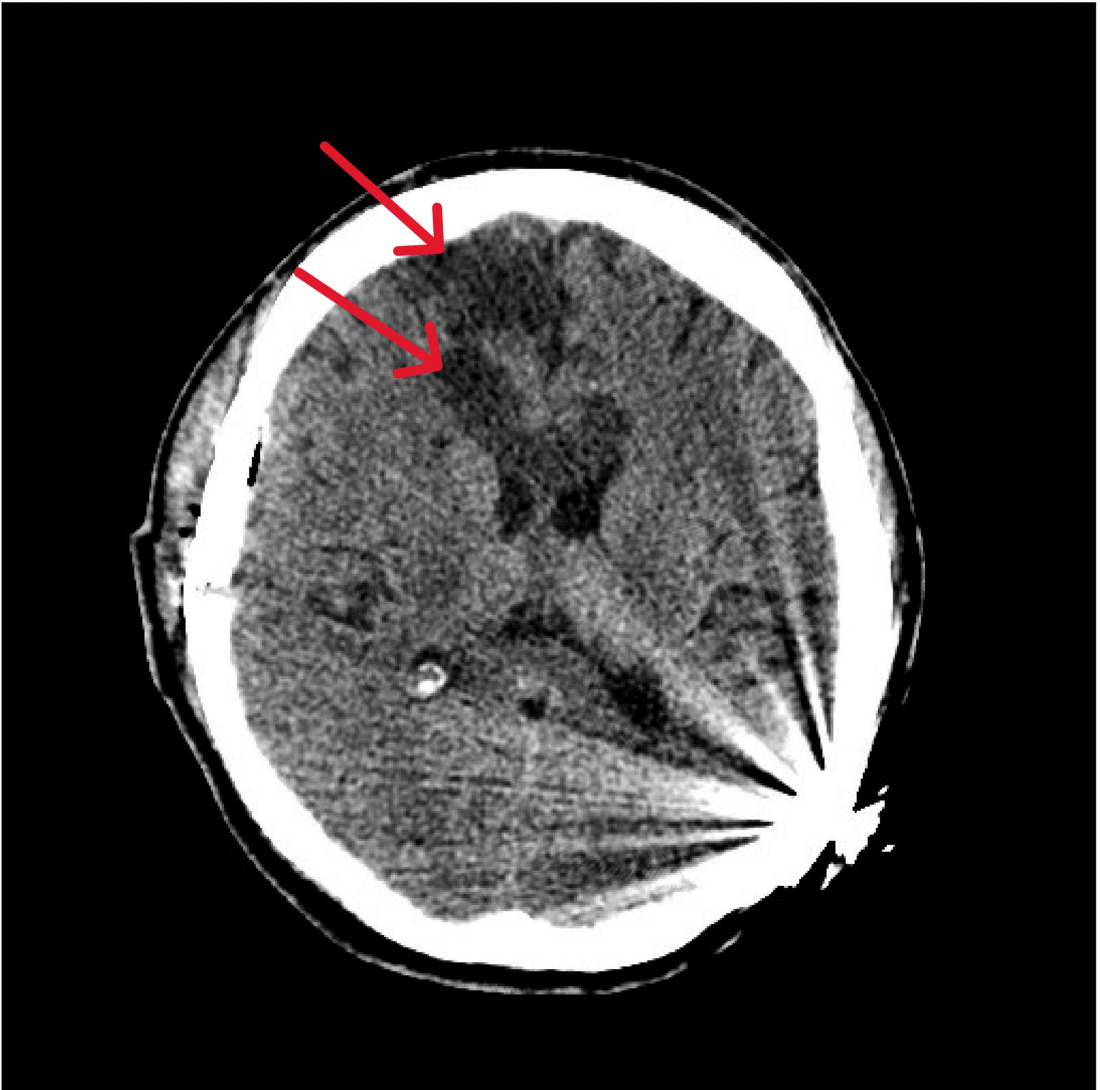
Follow-up postoperative CT imaging The image shows evolving infarction (red arrow) of the ACA and MCA territories without hemorrhagic conversion CT: computed tomography; ACA: anterior cerebral artery; MCA: middle cerebral artery
